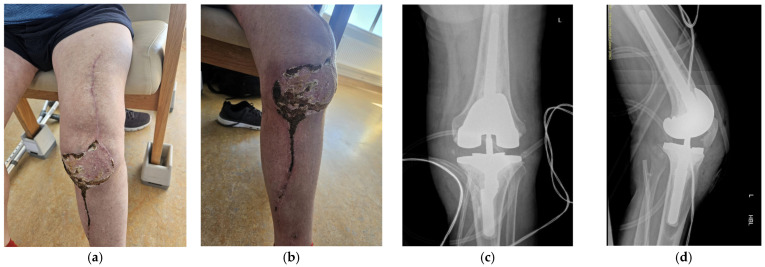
(a,b) Case 3 skin condition as of 10/04/25. (c,d) Plain films on 01/03/25 following second-stage revision.
